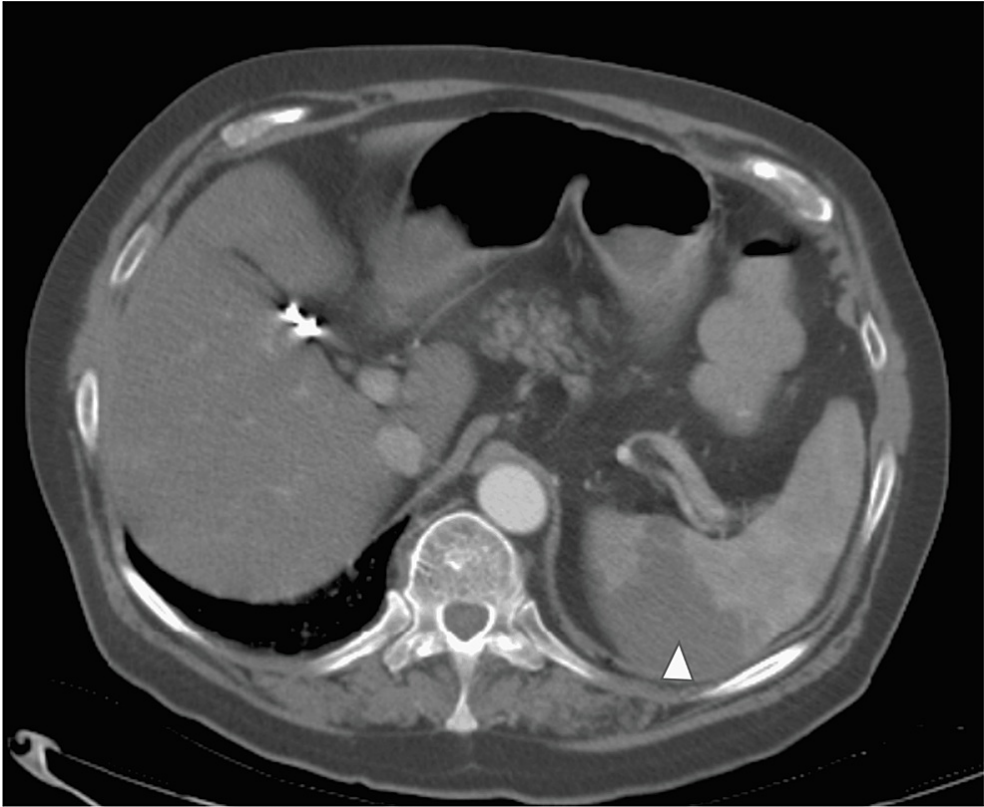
Computed tomography angiogram of the aorta showing hypoattenuation within the splenic artery for wedge-shaped areas around the hilum with possible infarction.
